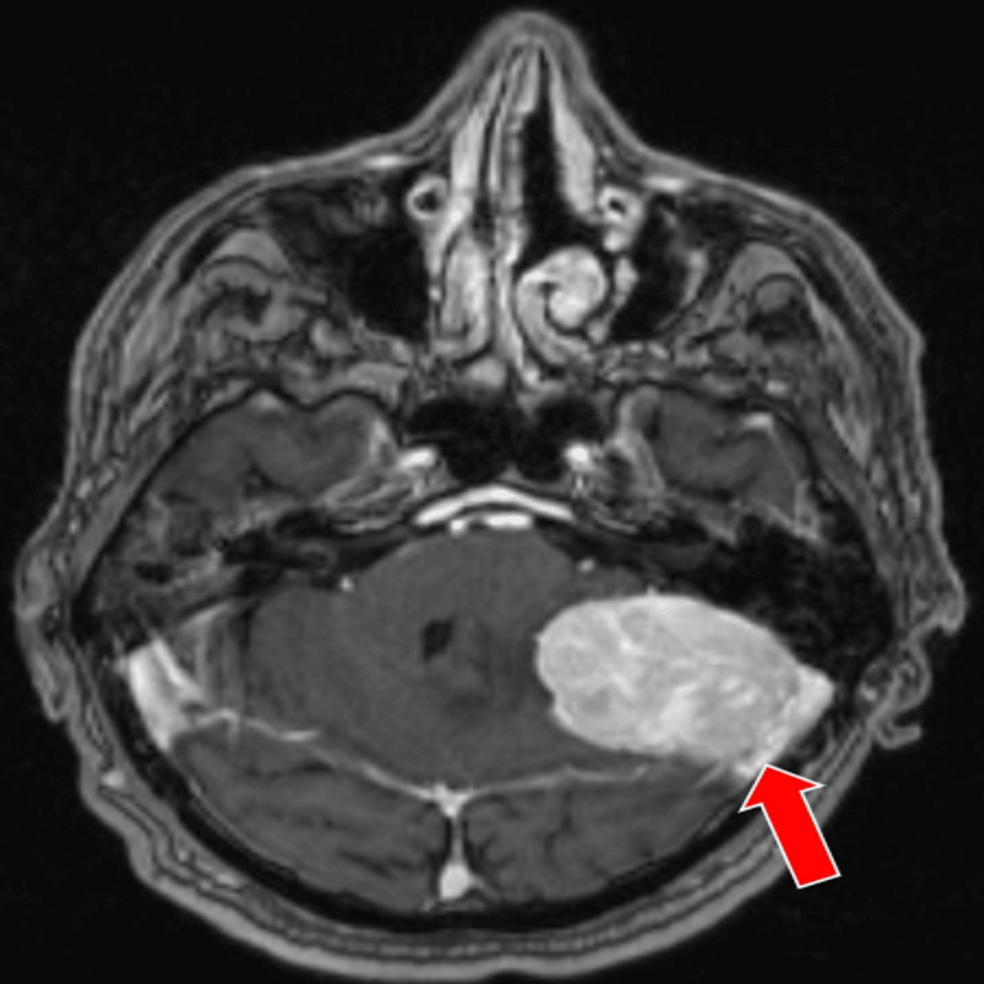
Preoperative MRI T1 MPRAGE sequence, axial section shows that tumor is hyperintense and it can be clearly delineated MPRAGE: Magnetization-Prepared Rapid Acquisition Gradient Echo
